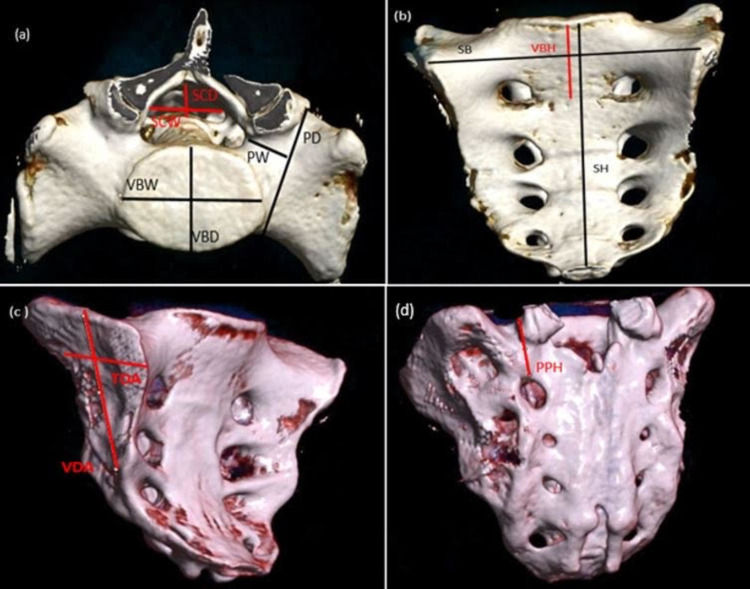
The MDCT shaded surface volume-rendering technique images of the sacrum with its morphometry obtained in a 256-slice CT scanner showing the various measurements. (a) the superior aspect of the sacrum: VBD at the S1 level, VBW, SCD, SCW, PD, and PW. (b) The anterior aspect of the sacrum: VBH at the S1 level, SB, and SH. (c) The lateral aspect of the sacrum: VDA and TDA. (d) The posterior aspect of the sacrum: PPH of the S1 vertebra. MDCT: multidetector computed tomography; VBD: vertebral body mid-diameter; VBW: vertebral body maximum width; SCD: spinal canal mid-diameter; SCW: spinal canal maximum width; PD: pedicle depth; PW: pedicle width; VBH: vertebral body mid-height; SB: sacral breadth; SH: sacral height; VDA: vertical diameter of the auricular surface; TDA: transverse diameter of the auricular surface; PPH: posterior pedicular height
